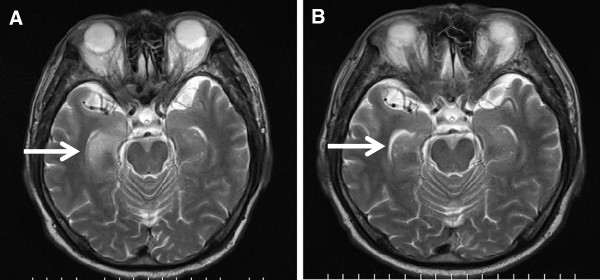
Imaging of brain magnetic resonance imaging at baseline and after chemotherapy. (A) Magnetic resonance imaging of the brain shows high signal intensity on T2-weighted image in the right temporal lobe (white arrow), with limbic encephalitis. (B) After one cycle of systemic chemotherapy, the high signal intensity was improved (white arrow).
